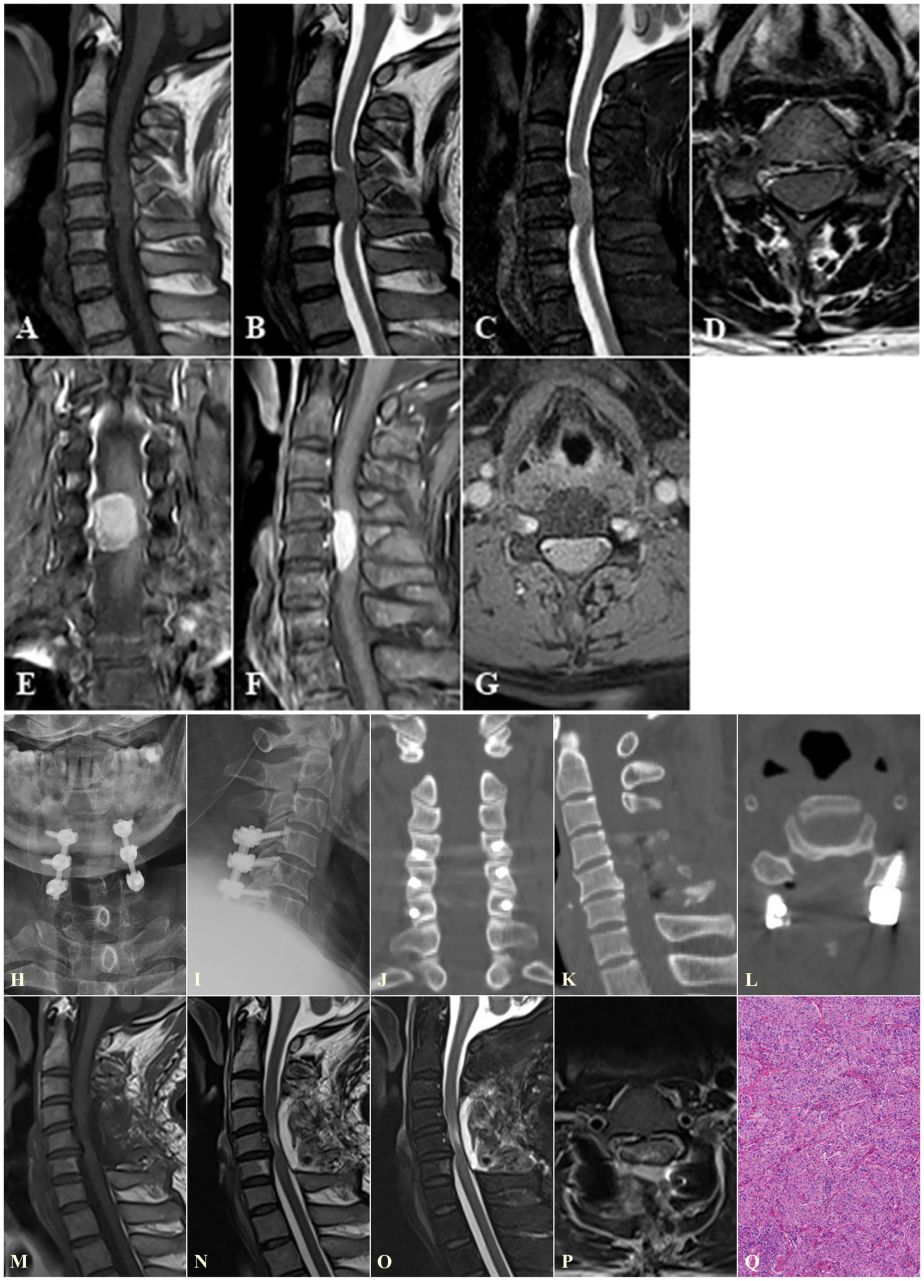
A representative case of one-piece resection for treating VIESM at C5. (A–G) Preoperative MRI images show the VIESM at C5. (H–Q) Postoperative X-ray (H, I), (J–L), MRI (M–P), and pathological (Q) images demonstrate the total removal of the VIESM via one-piece resection, and fine internal fixation. VIESM, ventral intradural extramedullary spinal meningioma; MRI, magnetic resonance imaging; CT, computed tomography.
